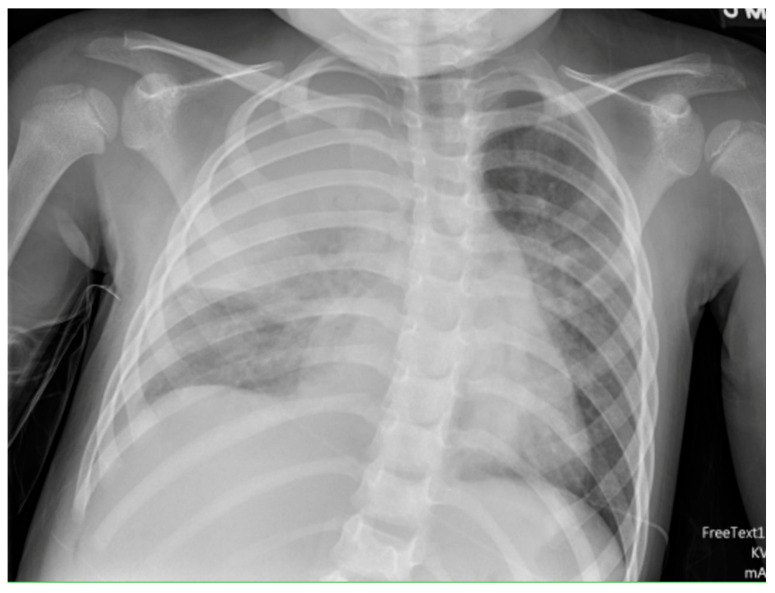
Necrotizing Pneumonia on Chest Radiograph. Plain chest radiograph showing consolidation in the right upper lobe.
